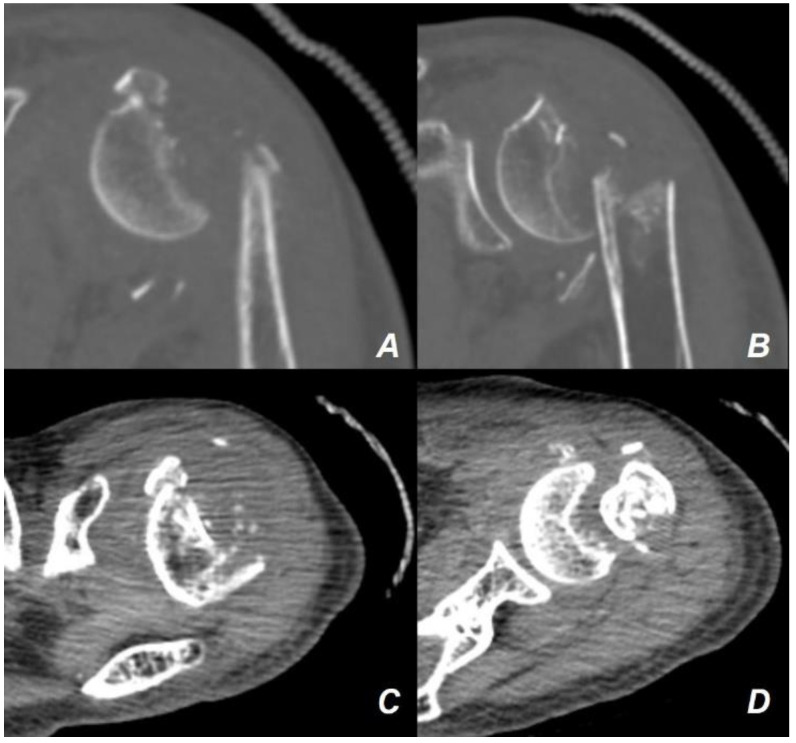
Computed tomographic (CT) slices of the injured arm. The CT slices of the injured arm showed a lesser tuberosity fracture of the proximal humerus, visible in both the coronal (A) and axial (C) planes. In addition, severe comminutions of the medial and lateral metaphysis areas can be identified in both coronal (B) and axial (D) planes of the CT slices.
